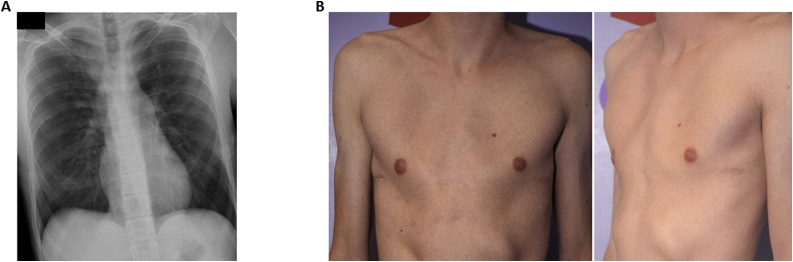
A, Anteroposterior chest radiograph after planned removal of final bar. B, Postoperative photograph of chest.
